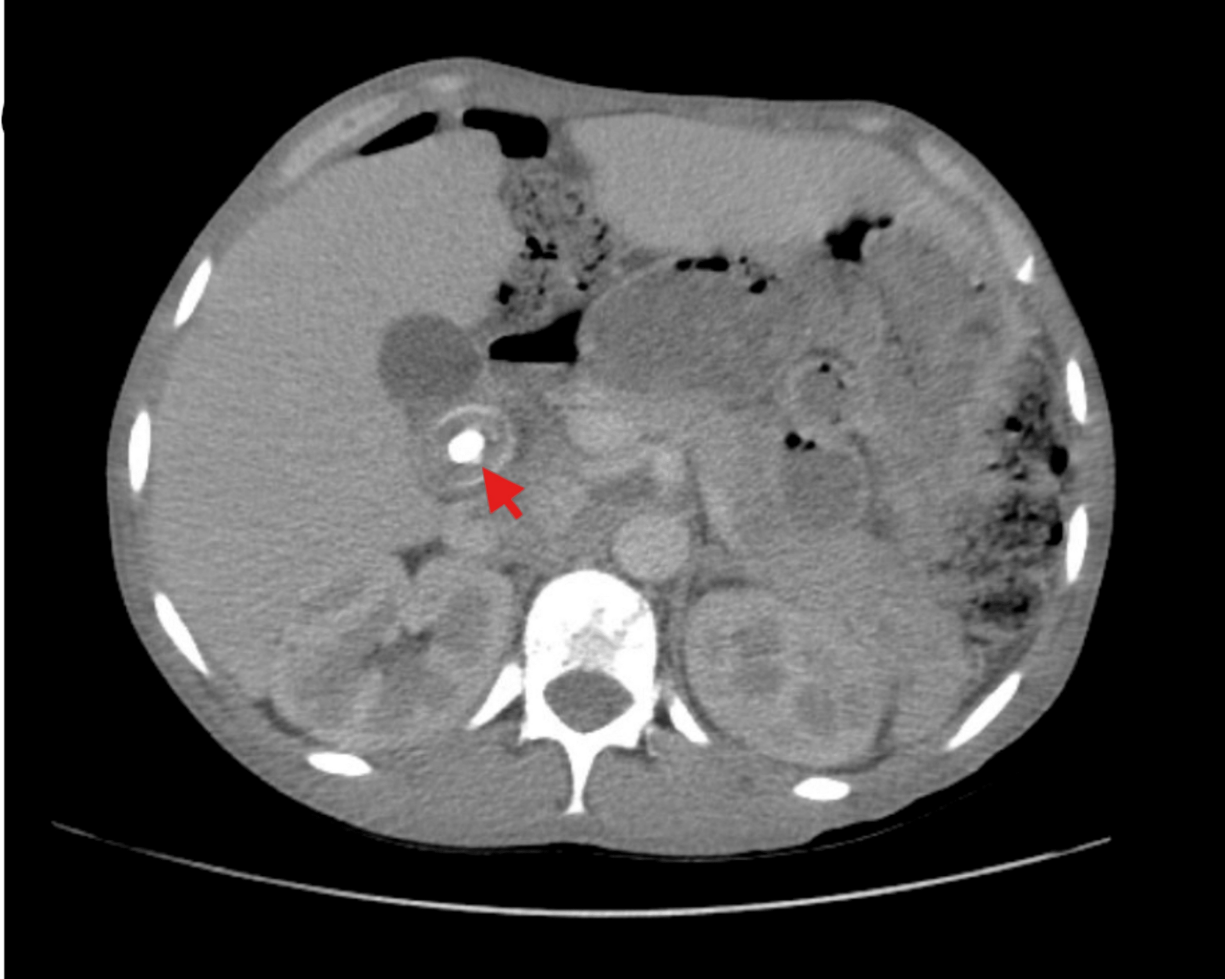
Axial view of computed tomography of abdomen revealing distended gallbladder with calculus (red arrow) measuring 3.1 x 2.1 x 2.2 cm impacted in neck of the gallbladder (case 3).
